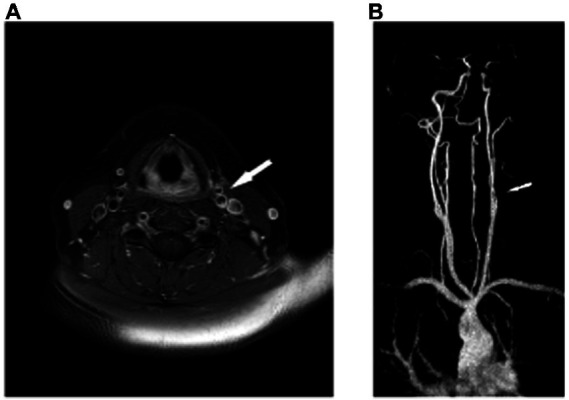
(A) Postoperative MR T1-weighted axial image of the neck with fat suppression after contrast medium administration at the level of CCA bifurcation with evidence of no mass. (B) Postoperative contrast contrast-enhanced MR angiography (CE-MRA) of the neck, maximum intensity projection (MIP) reconstruction in the coronal plane with evidence of no pathological vascularization. Only mild irregularities of CCA branches were demonstrated with no significant stenosis.
